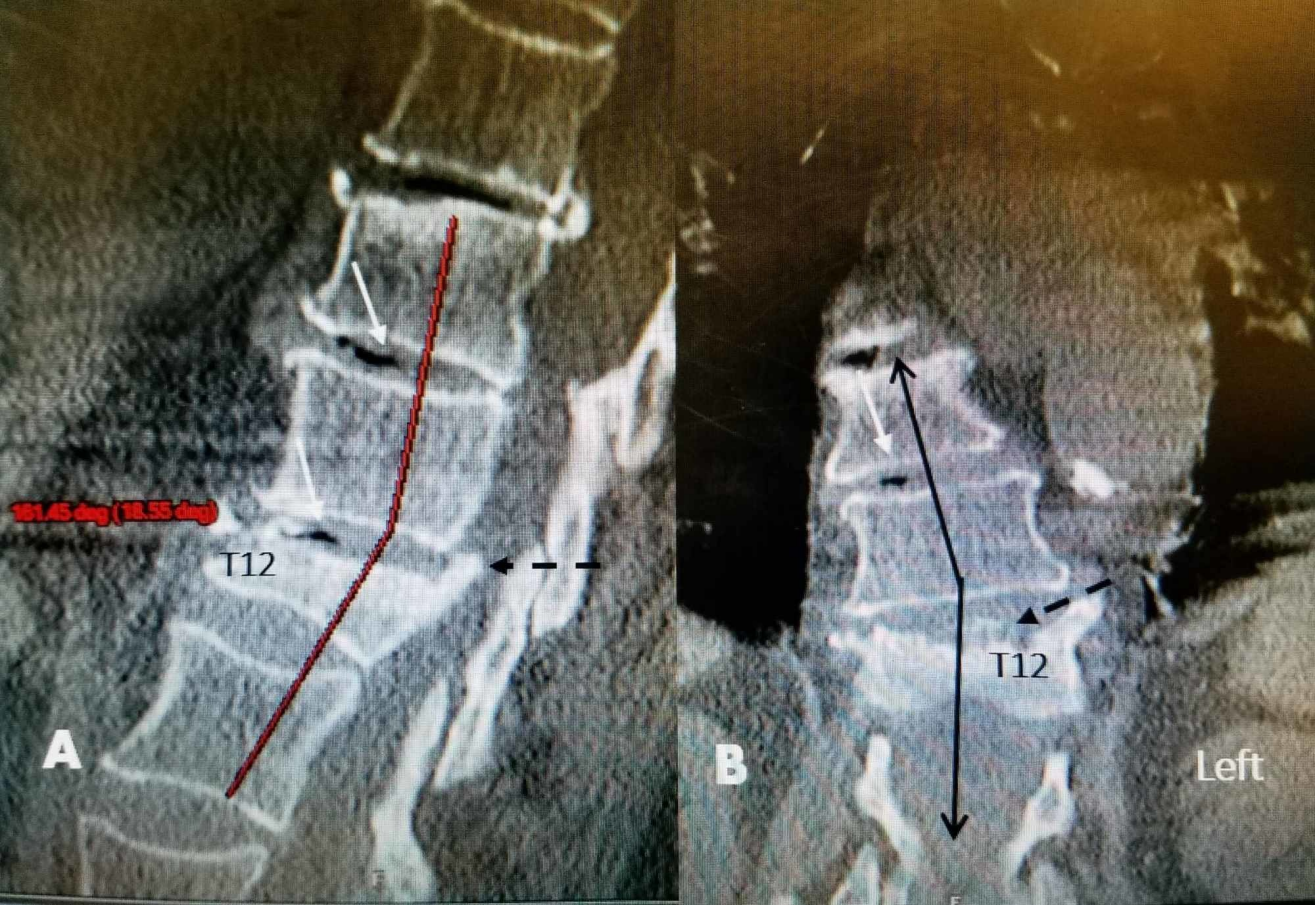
CT scan before initial kyphoplasty A: initial sagittal computerized tomography (CT) scan showing collapse of T12 with 10 degrees of kyphotic angulation (solid line) with dorsal displacement of the superior endplate of T12 into the spinal canal (dashed black arrow). B: coronal CT scan showing 10-degree angulation to the right of T11-T12. There is asymmetric collapse of the superior endplate of T12 more on the left side (dashed black arrow) CT: computed tomography
